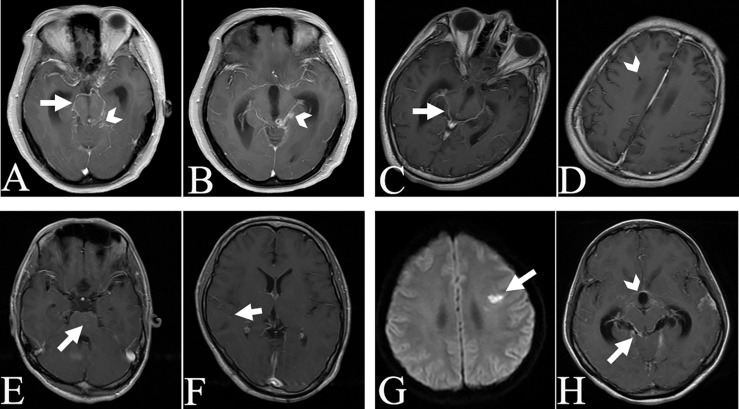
Magnetic resonance imaging images of selected TBM cases. Basicranial meningeal enhancement (arrow) and tuberculoma (arrowhead) in case 4 (A,B). Basicranial meningeal enhancement (arrow) and lateral ventricular drainage tract (arrowhead) in case 5 (C,D). Miliary tuberculous foci (arrow) in case 5 (E,F). Infarction focus (arrow, G), meningeal enhancement (arrow, H) and enlarged third ventricle of cerebrum (arrowhead, H) in case 6.
